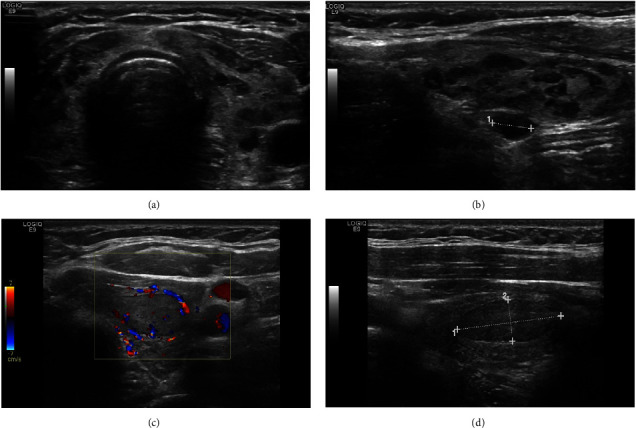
Ultrasound images for (a) and (b) diffuse lymphocytic thyroiditis with giraffe appearance. (c) and (d) Focal lymphocytic thyroiditis appears as a solid hypoechoic nodule (TR4).
